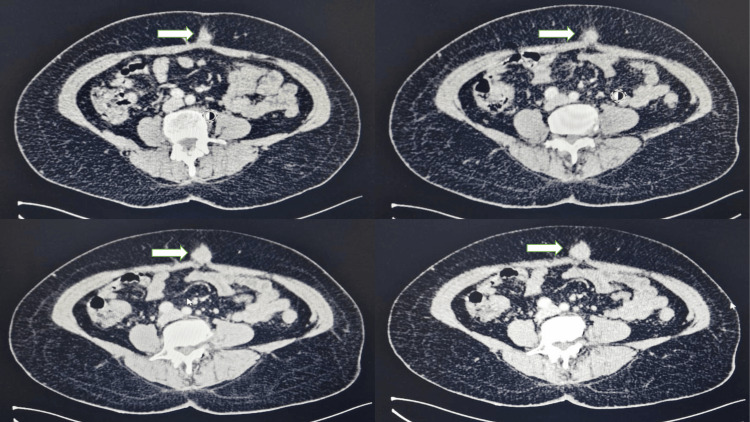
A computed tomography scan revealed the presence of an umbilical nodular formation, oval-shaped, hypodense, and enhanced after contrast injection (arrow), suggestive of a Sister Mary Joseph nodule.
